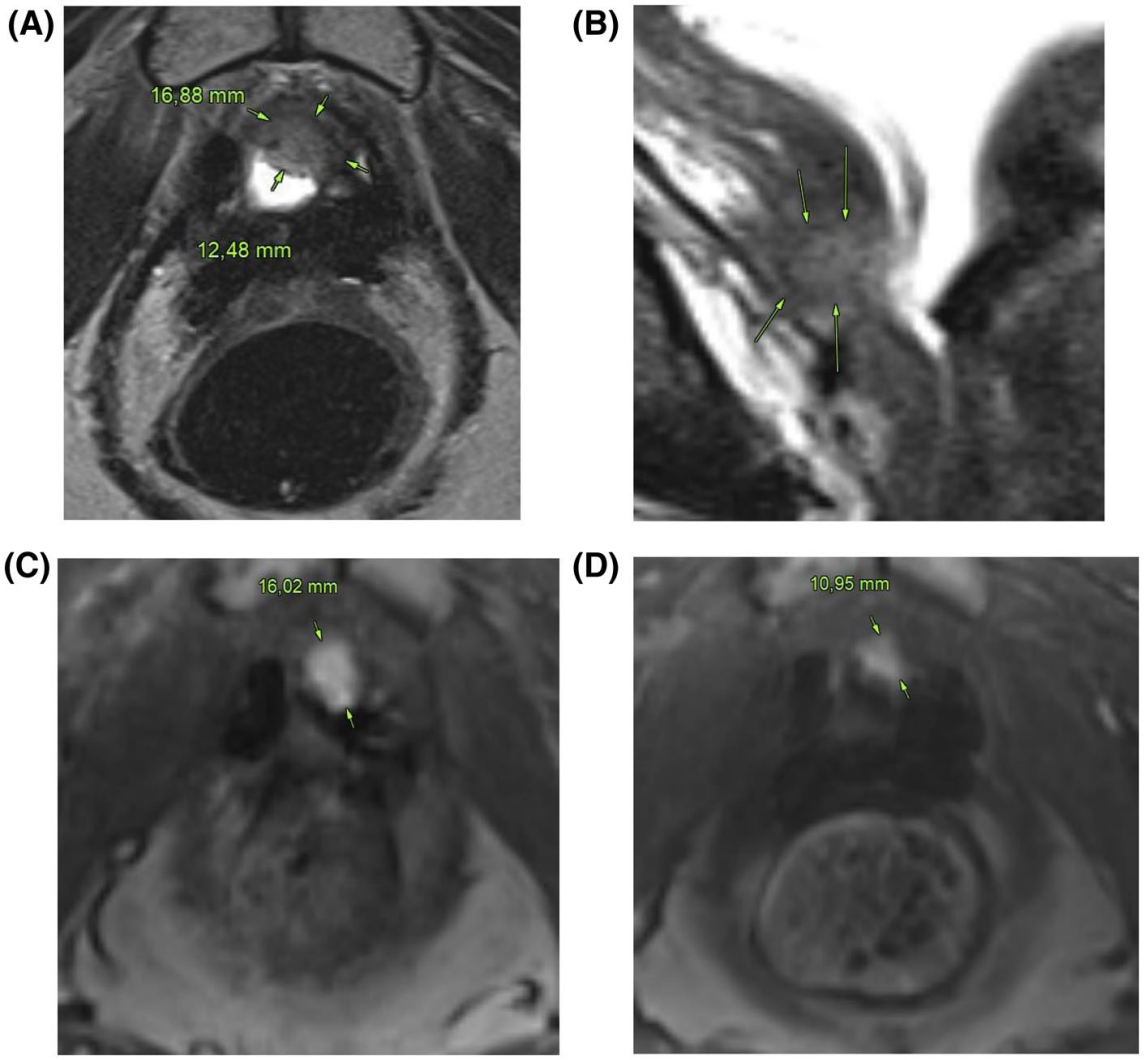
High‐resolution 3D SPACE 3 Tesla MR image shows a 17 × 12 mm soft tissue mass at the ventral aspect of the anastomosis, consistent with local tumor recurrence after radical prostatectomy (A). A sagittal T2w image demonstrates the anatomic relationship of the lesion to the bladder wall and the urogenital diaphragm (B). Pre SABR (C), the lesion is markedly hypervascular on a single transaxial arterial phase MR perfusion image measuring 16 mm in its greatest diameter; 6 months after treatment (D), vascularity of the lesion is significantly reduced and the size of the enhancing portion of the mass has shrunk to 11 mm consistent with response to the treatment
